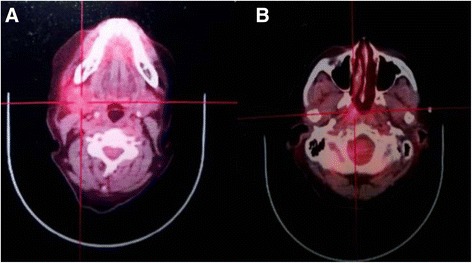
PET/CT revealed high FDG uptake in a surgical regions and b ipsilateral nasopharynx
